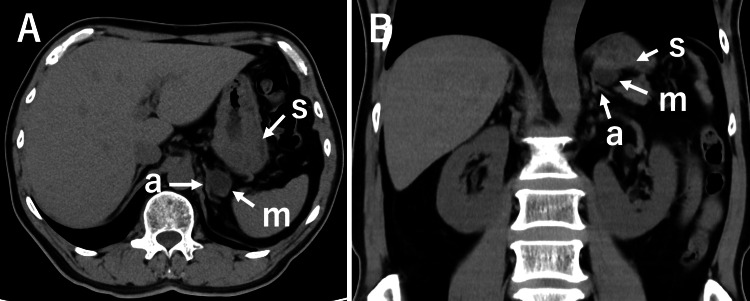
Axial (A) and coronal (B) cross-sectional CT images. The letters s, a, and m denote the stomach, left adrenal gland, and a mass shadow, respectively. A mass (m) is observed adjacent to the stomach (s) and the left adrenal gland (a).
